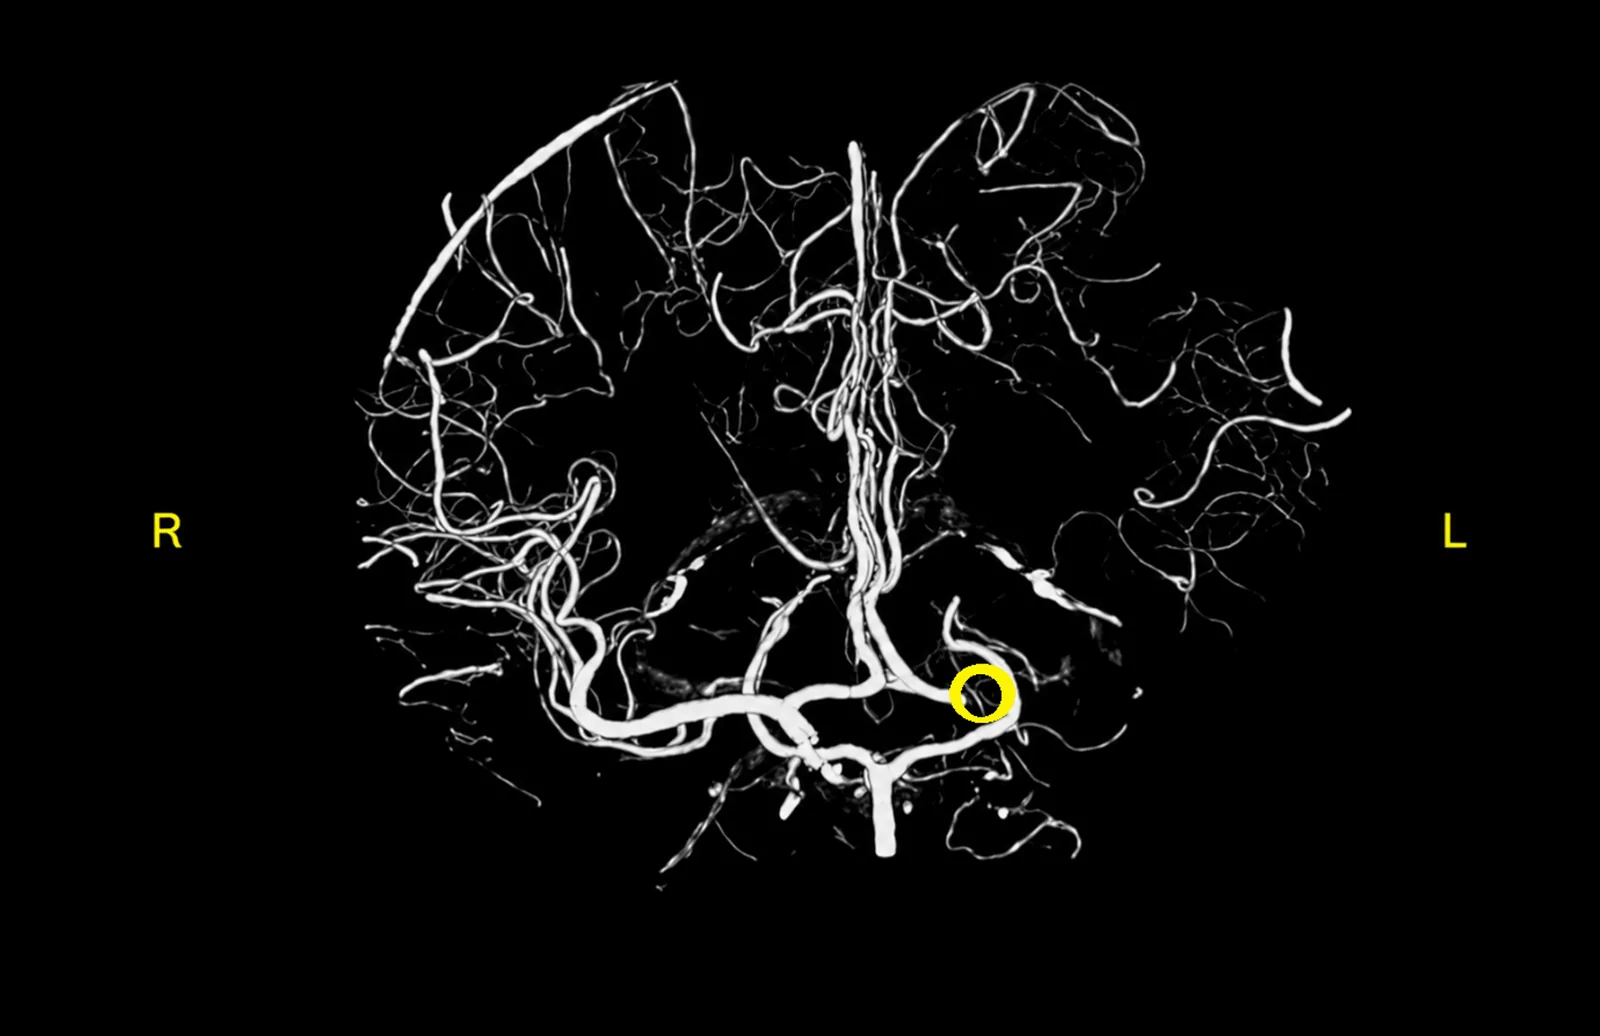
CT cerebral angiography Repeat vascular imaging following recurrent neurological deterioration approximately one week after the index stroke, demonstrating a new left M1 middle cerebral artery occlusion (yellow circle).
